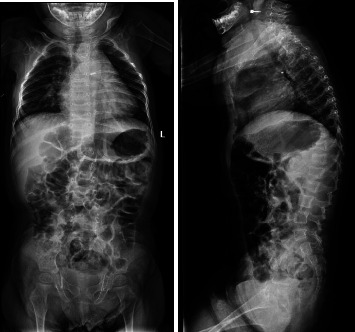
Radiographs at 1 year of age.
